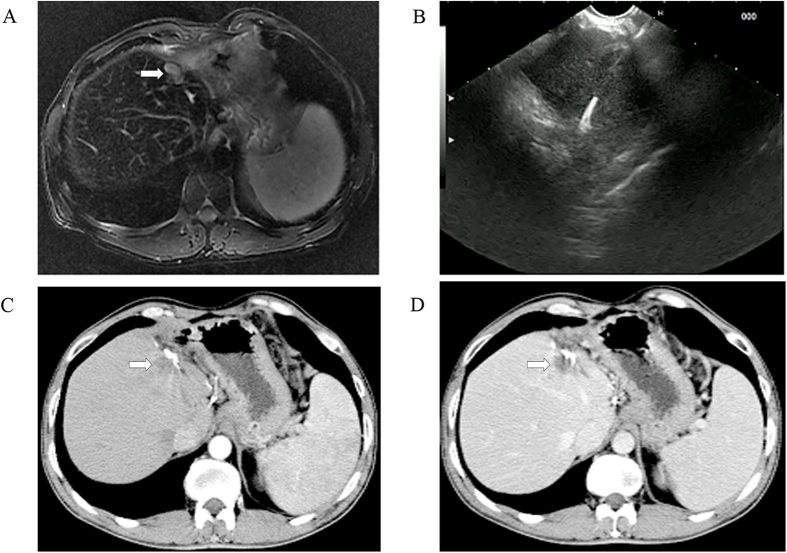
EUS-guided anhydrous ethanol ablation for postoperative liver recurrence of hepatocellular carcinoma. (A) presence of a 1.5 cm × 1.1 cm postoperative liver recurrence located on the resection margin as shown on T_2_-weighted MRI scans (white arrows), but conventional US image failed to show the lesions; (B) EUS-guided ethanol injection; and (C) arterial phase (arrowhead) and (D) parenchymal phase (arrowhead): non-enhanced liver disease on follow-up contrast CT scan revealed complete resolution of disease at 12 months.
